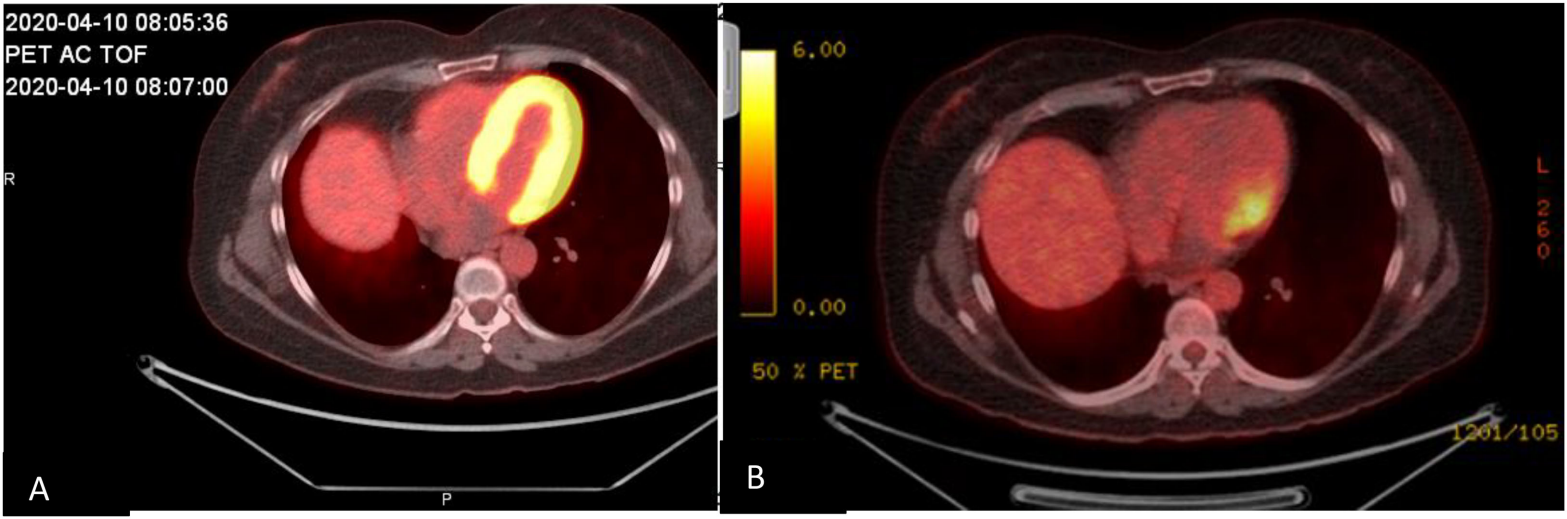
Cardiac positron emission tomography/computed tomography images obtained (A) during initial hospitalization, showing FDG activity surrounding the myocardium, and (B) 4 months after hospitalization, showing improvement of FDG activity surrounding the myocardium.
